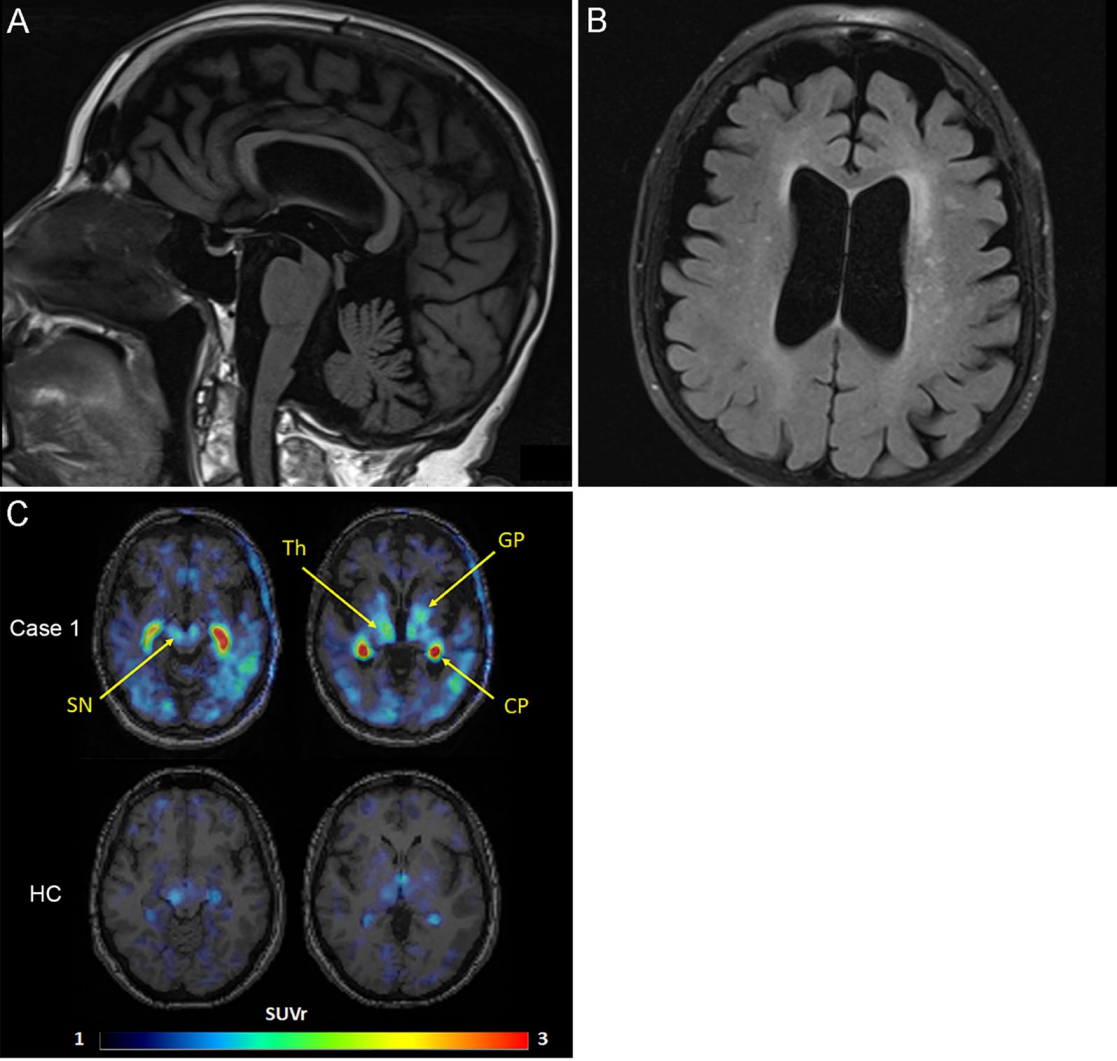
Representative images of brain MRI and tau PET. A The sagittal T1-weighted FLAIR image demonstrates atrophy of the midbrain. B The axial T2-FLAIR image displays asymmetrical cerebral atrophy with left-sided predominance, white matter hyperintensities, and dilation of the lateral ventricles. C Representative images of [^18^F]APN-1607 of Case 1 and a 54-year-old healthy female (HC). [^18^F]APN-1607 uptake is moderately increased in the globus pallidus, thalamus, and substantia nigra (indicated by arrows), compared to the HC. The two intense spots represent signal in choroid plexus (CP), which is non-specific and is occasionally seen in some patients and controls. The SUVr intensity color scale is shown at the bottom
